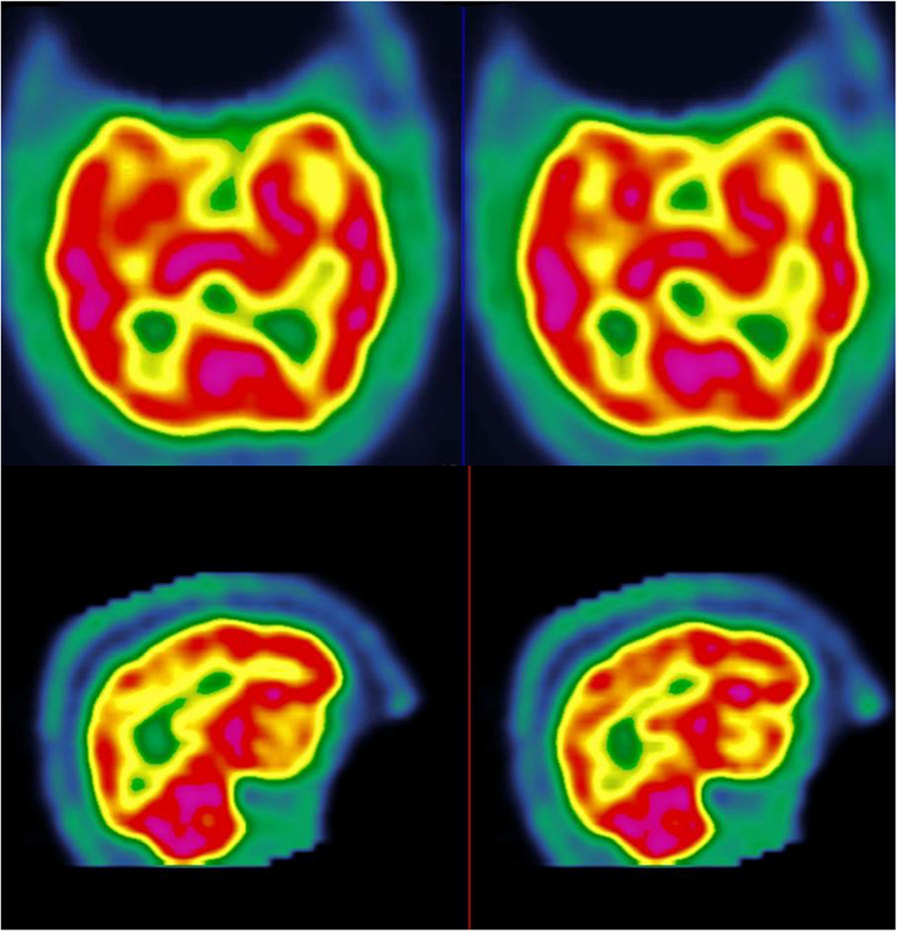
Results from the second SPECT (after the cessation of lenalidomide treatment). Note the normal perfusion in the transverse (top) and sagittal (bottom) slices.
